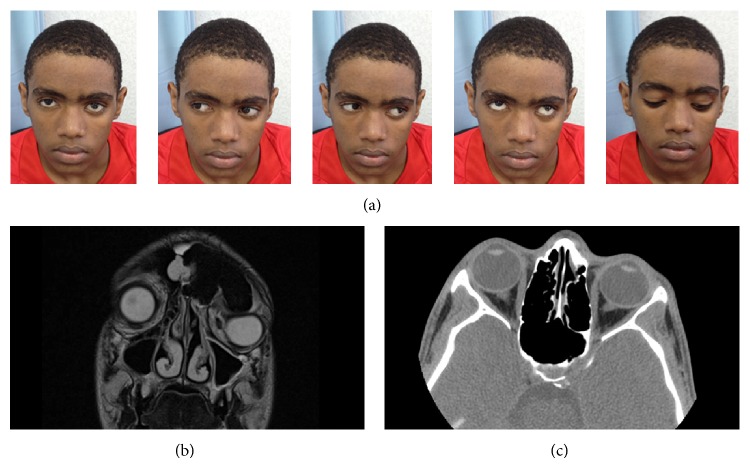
Postoperative patient photo demonstrating the resolution of proptosis and fulleye movements (a). Preoperative coronal MRI orbit showing the degree of compression on the orbit (b). Postoperative CT orbit showing the resolution of proptosis (c).
